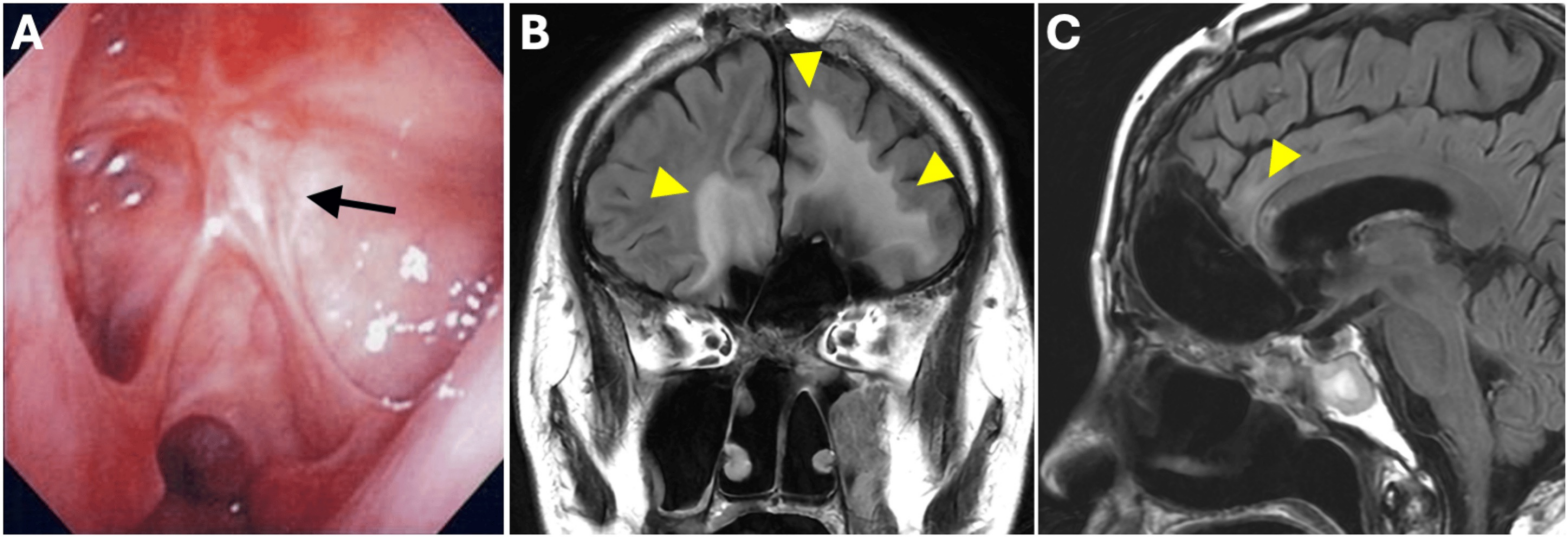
Findings 12 years after the surgery. (A) Nasal endoscopy revealed no evidence of local recurrence in the nasal cavity (black arrow). (B, C) Gadolinium-enhanced T1-weighted magnetic resonance imaging revealed edematous changes consistent with prior radiotherapy (yellow arrowhead) but no obvious evidence of tumor recurrence.
